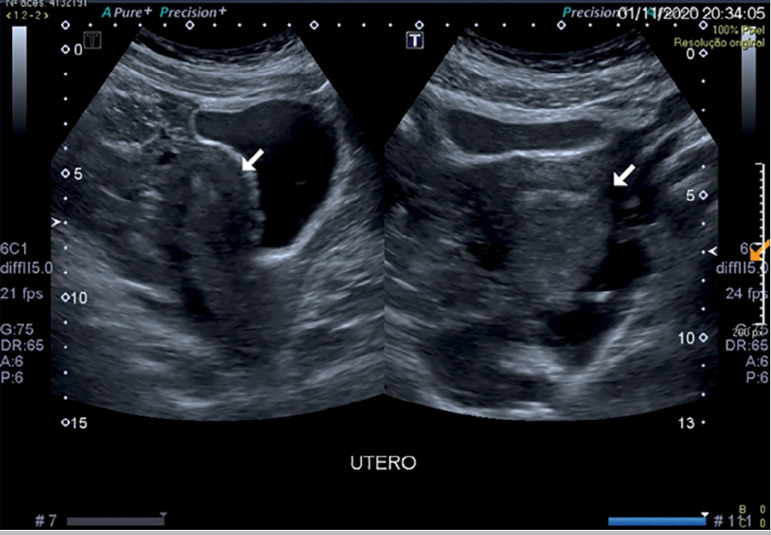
Pelvic ultrasound 12 hours after oocyte retrieval.
